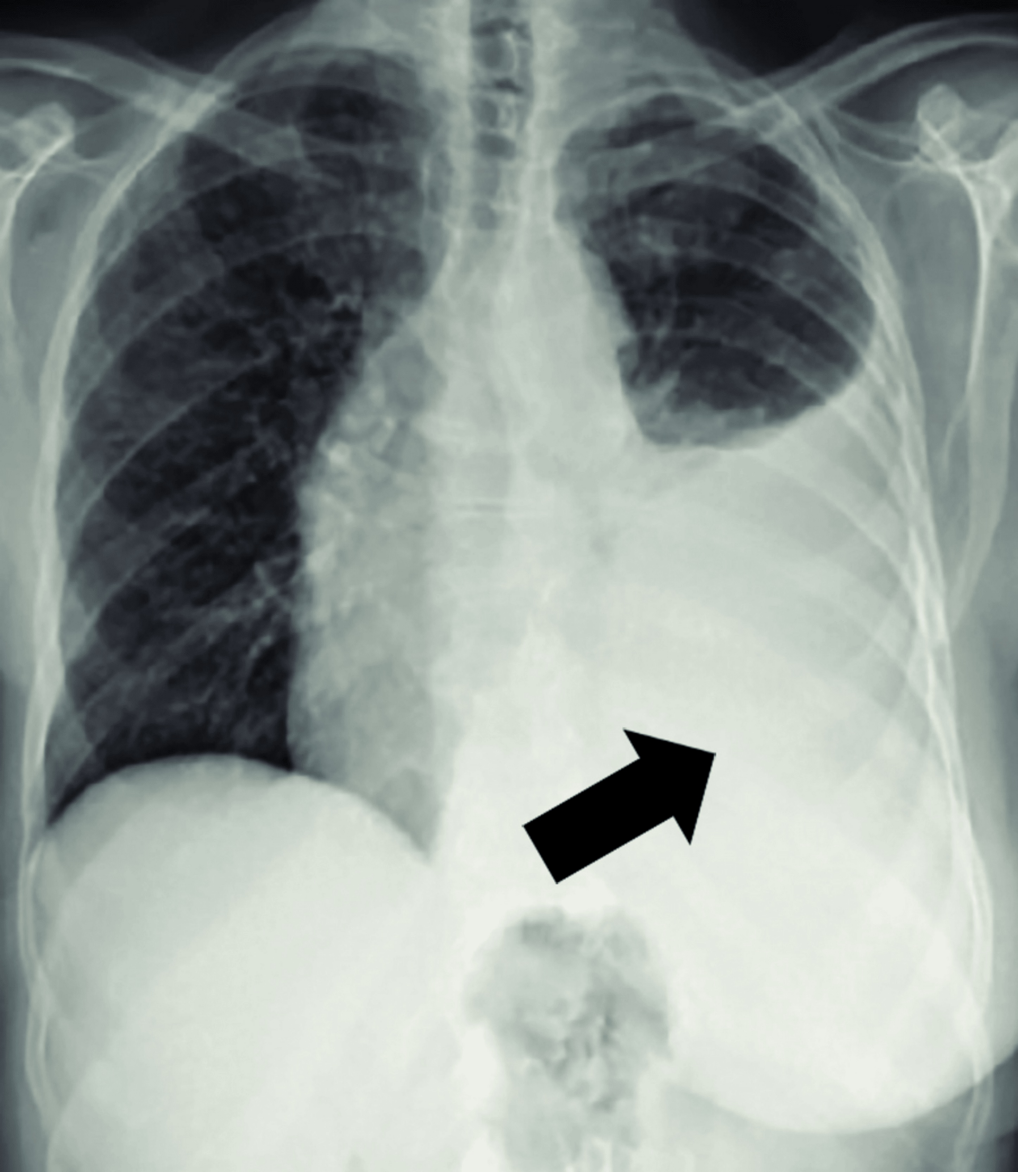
Chest X-ray showing mild left lower lobe pleural effusion (indicated by arrow) consistent with serositis in systemic lupus erythematosus.
